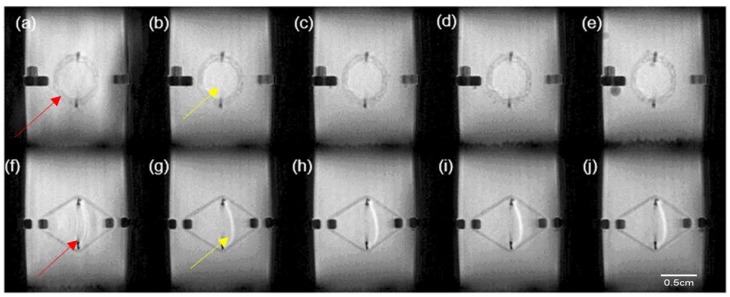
MRI images of the PPP scaffold from the front view at (a) 0 h; (b) 2 h; (c) 4 h; (d) 8 h; (e) 24 h and from side view at (f) 0 h; (g) 2 h; (h) 4 h; (i) 8 h; and (j) 24 h, where red arrows depict the PCL scaffold and yellow arrows depict the PVA–PAA hydrogel.
